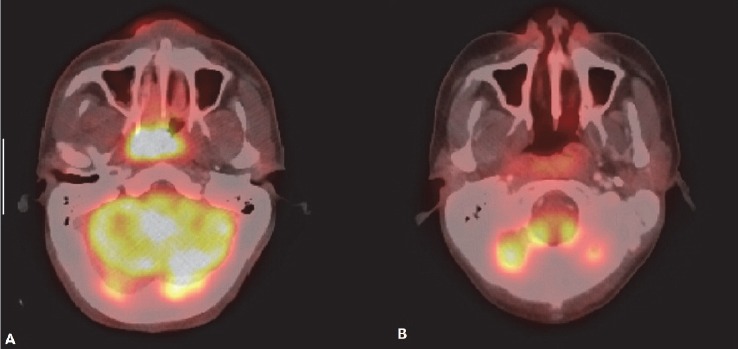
NHL of nasopharynx: (A) FDG avid soft tissue mass is seen in right posterolateral wall of the nasopharynx with obstruction of right posterior nasal choana. (B) Post chemotherapy scan for response assessment shows complete metabolic response
